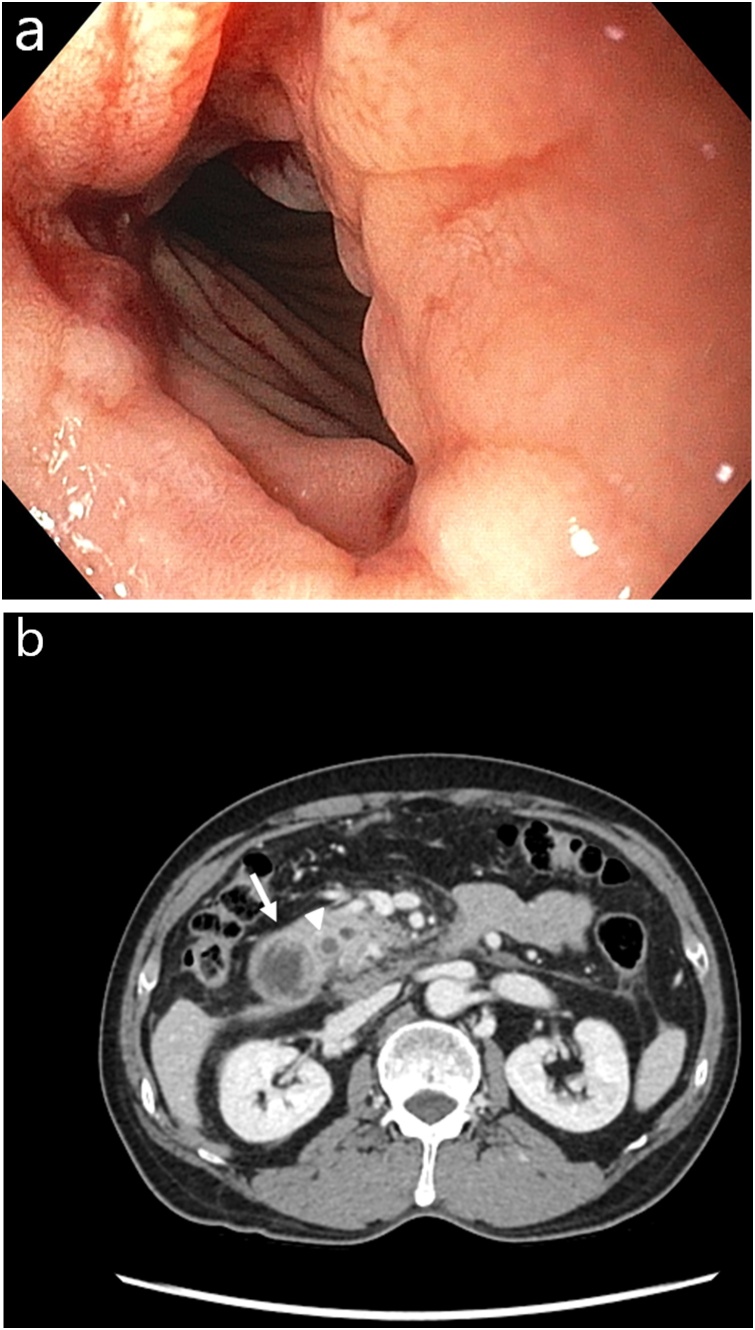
Preoperative imaging features. (A) Esophagogastroduodenoscopy image showing luminal stenosis and edematous changes affecting the second and third parts of the duodenum, though the involved lumen is not obstructed completely. (B) Computed tomography scan of the patient’s abdomen and pelvis showing faintly enhanced thickening of the involved duodenal walls (arrow) along with mild dilatation of the common bile duct (arrowhead).
